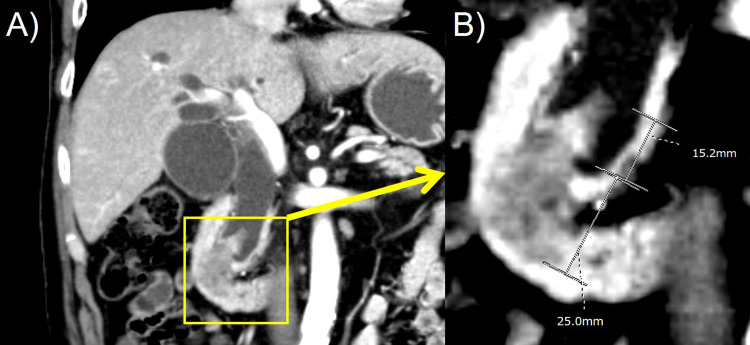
Radiological findings of tumors of the ampulla of Vater (AoV) and common bile duct (CBD) (A,B). The dynamic CT study in an artery-dominant phase indicates that the mass consists of two components: a hypovascular, 2.5 cm-sized exophytic tumor component in the AoV and a hypervascular, 1.5 cm-sized polypoid tumor component in the lower part of the CBD.
